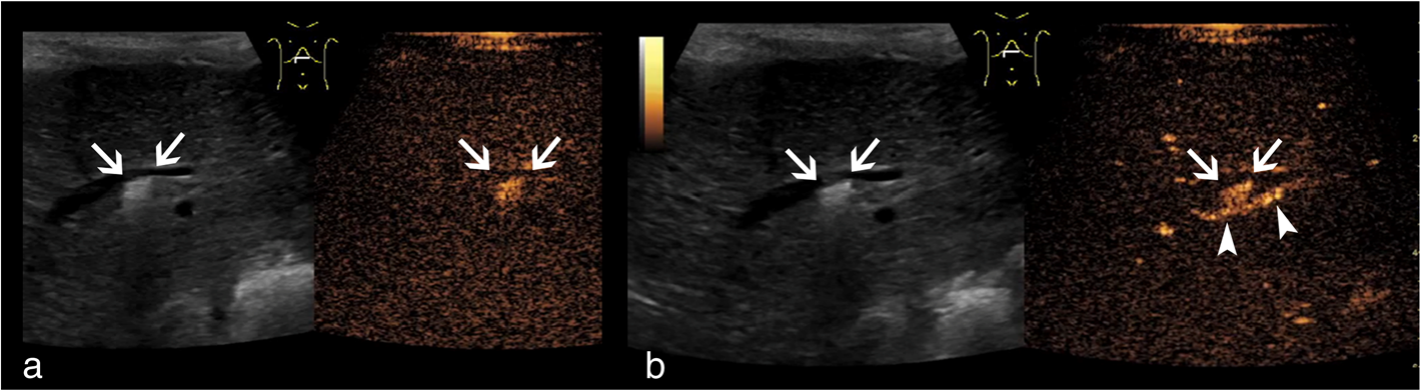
Post-interventional sonography displayed in contrast mode with split-screen; B-mode images are visible on the left, and contrast-mode images on the right side respectively. a Post-interventional ultrasound before contrast medium injection detects artifacts of coils (arrows) on B-mode (left side) contrast-mode images (right side). b Post-interventional contrast-enhanced sonography during arterial phase shows coils artifacts without contrast-enhancement of the pseudoaneurysm (arrow) and patency of the SIII artery (arrow heads) on B-mode (left side) and contrast-mode imaging (right side)
